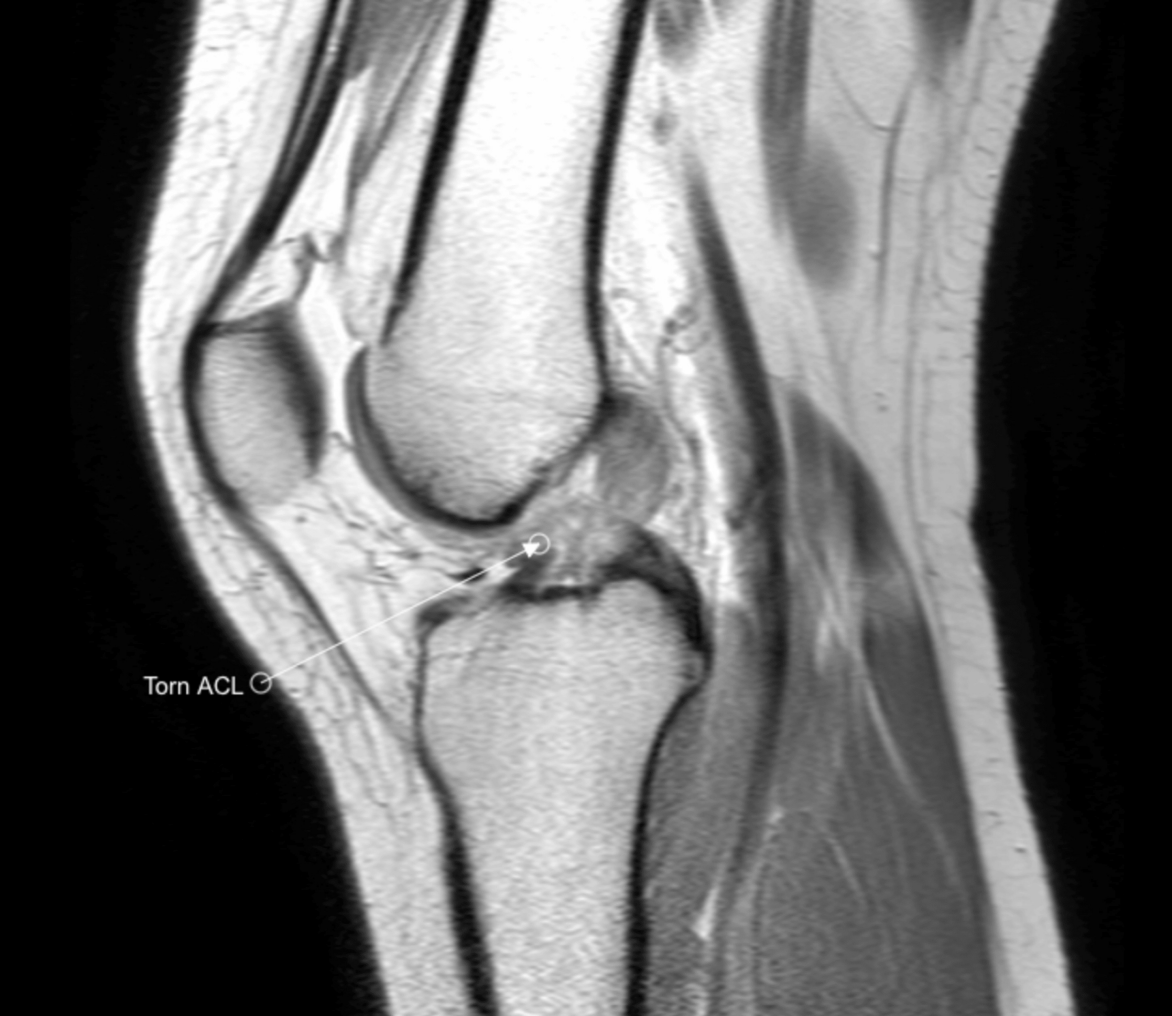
PD MRI sagittal view of the left knee showing torn ACL PD: Proton density; ACL: Anterior cruciate ligament.
